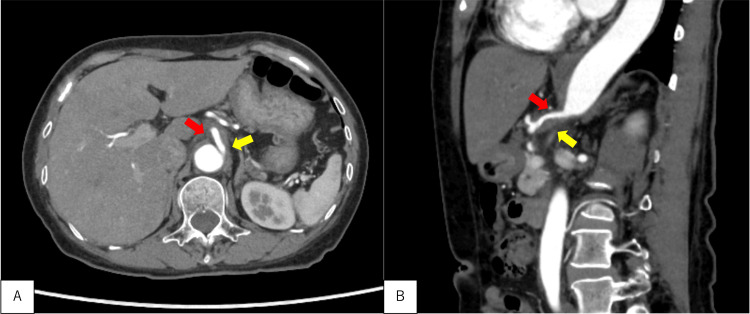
Contrast-enhanced CT images Contrast-enhanced CT (horizontal (A)/sagittal (B) plane) showing a thrombosed dissection of the celiac artery with narrowing of the luminal diameter; other major abdominal arteries remain intact (red arrow: true lumen, yellow arrow: false lumen) CT, computed tomography
